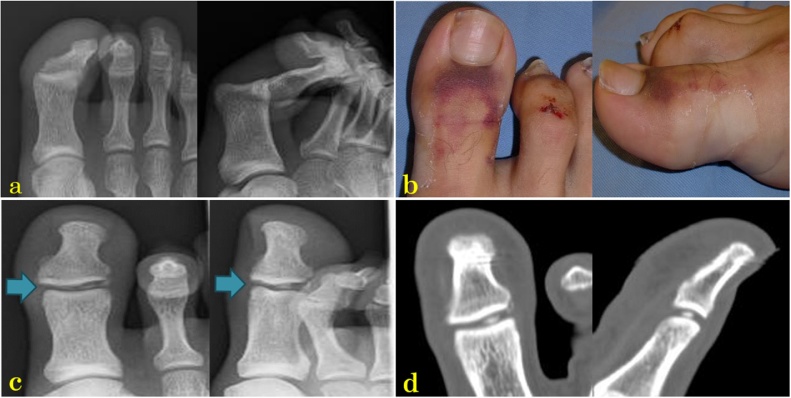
(a). X-rays during the first medical examination showing the posterior dislocation of the hallux interphalangeal (IP) joint. (b) After closed reduction, there was no macroscopic clear abnormality, but the joint cannot be moved. (c) The IP joint expanded a little, and a sesamoid spicule was noted in the IP joint. (d) Computed tomography showing the sesamoid spicule in the IP joint.
